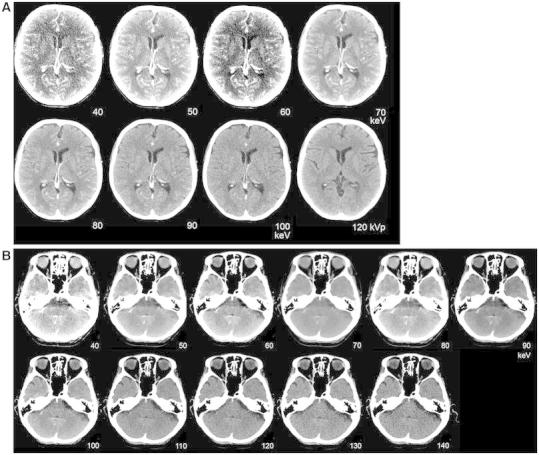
Monoenergetic reconstructions derived from a DECTV at the level of the supratentorial white matter/basal ganglia and the posterior fossa. SECT at 120 kVp is added for comparison. At lower monoenergies the SNR is lower, with higher CNR. Optimum level in the supratentorial brain is between 50 and 70 keV. At lower energies the beam-hardening artifacts in the posterior fossa are more pronounced, whereas they decrease at higher energies. Optimal imaging requires higher energies for posterior fossa compared to supratentorial brain (conventional CT 120 kVp, 285 mAs, CTDI 48.28 mGy, DLP 666 mGycm. DECTA 80/Sn140 kVp, 310/155 mAs, CTDI 28.86 mGy, DLP 458 mGcm. 90 cc iodinated contrast 300 mg/ml; injectionrate 3 cc/s; 40 cc saline flush 3 cc/s, delay 45 s)
